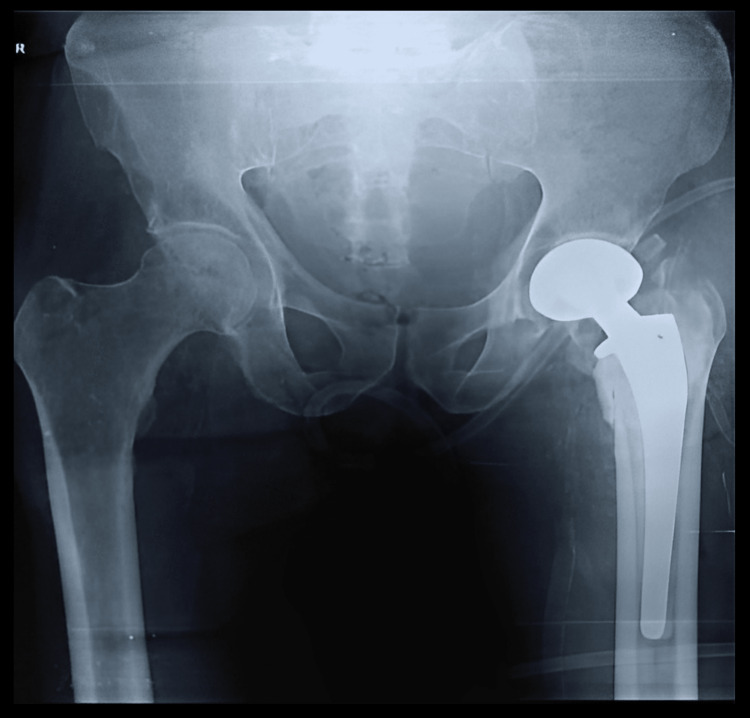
Postoperative anteroposterior X-ray view of the same patient managed by primary cemented bipolar hemiarthroplasty
